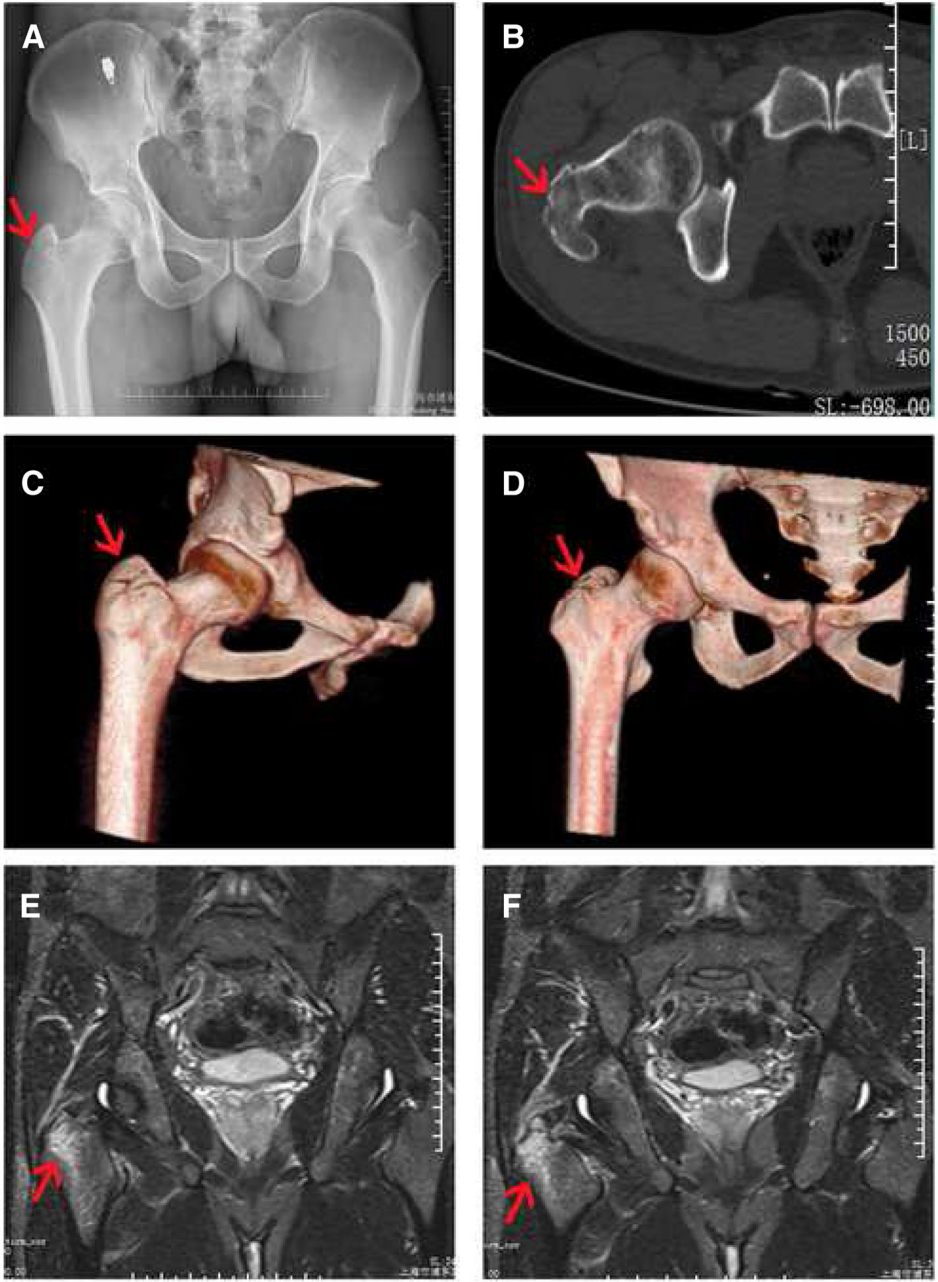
A 45-year-old man injured in a traffic accident presented with right hip pain. a AP radiograph showing only a minimally displaced isolated fracture of GT (red arrow). b, c, d CT and three-dimensional reconstruction image revealed a non-displaced greater trochanteric fracture (red arrow). e, f MRI revealed a fracture from the GT leading toward the lesser trochanter (red arrow)
